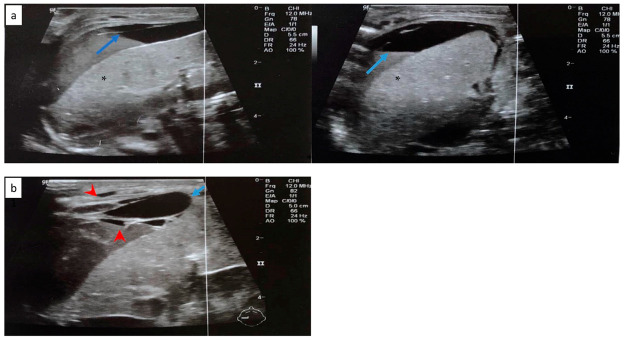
(a) Free perihepatic fluid collection (blue arrow) in abdominal ultrasound. (b) Abdominal ultrasound depicting subcapsular hematoma of the liver (blue arrow) and hemorrhagic collections in the peritoneal cavity (red arrowhead). Normal liver parenchyma is noted with asterisk (*).
